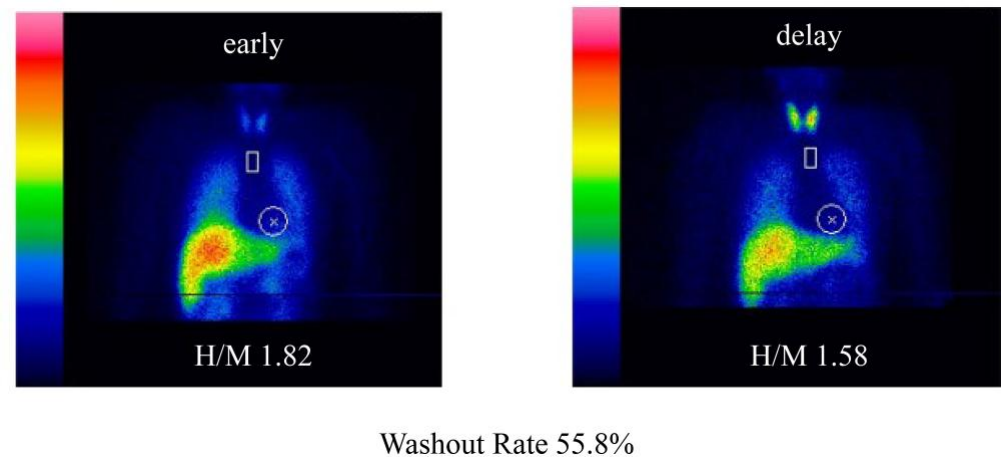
123-I-meta-iodobenzyguanidine (MIBG) myocardial scintigraphy performed on day 48. The image shows decreased cardiac uptake with a heart-to-mediastinum (H/M) ratio of 1.82 (early value) and 1.58 (delayed value), along with an increased washout rate.
